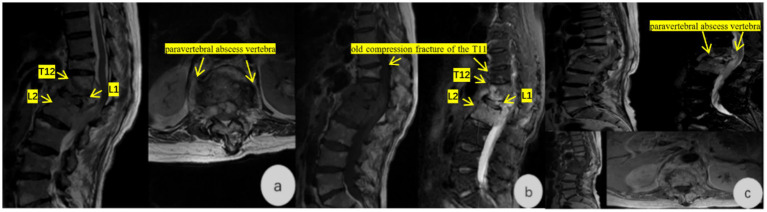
(a,b) Thoracolumbar spine MRI: Infectious lesions in the T12–L2 vertebral bodies with paravertebral abscess formation (dorsal and ventral); L1 vertebral body pathological fracture with mild spinal canal stenosis at the T12–L2 level; old compression fracture of the T11 vertebra. (c) Contrast-enhanced thoracolumbar spine MRI: Persistent infectious lesions in the T12–L2 vertebral bodies with reduced paravertebral abscess size (≈50% reduction compared with mid-June).
